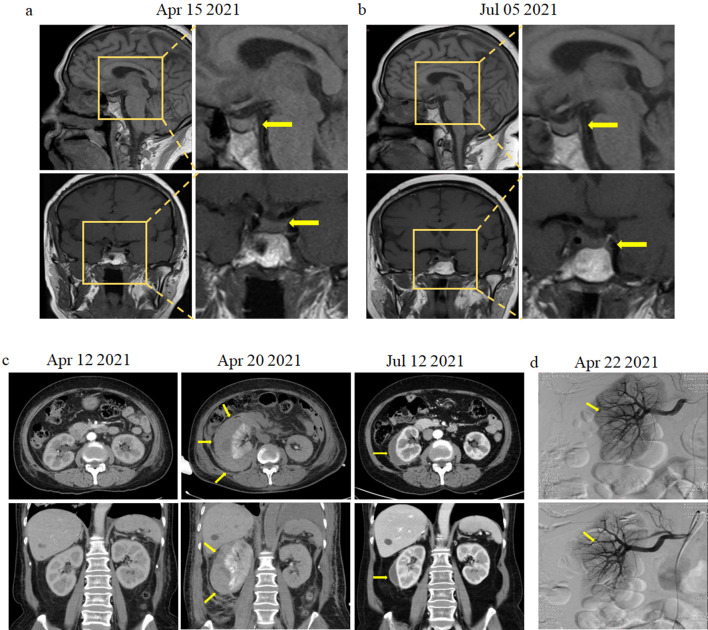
Magnetic resonance imaging (MRI), computed tomography (CT), and arterial angiography images of the patient. (a) Sagittal and coronal pituitary T1-weighted MRI showing local signal enhancement (yellow arrow). (b) Sagittal and coronal pituitary T1-weighted MRI showing normal signal (yellow arrow). (c) Abdominal CT scan showing a large right-sided perinephric and intracapsular hematoma in the right kidney (yellow arrow). (d) Arterial angiography showing active bleeding from the superior segmental and interlobular renal artery of the right kidney. Disseminated arterial microaneurysms are indicated by a yellow arrow (upper panel). Highly selective arterial embolization of the interlobular artery of the right kidney using microcoils (lower panel).
